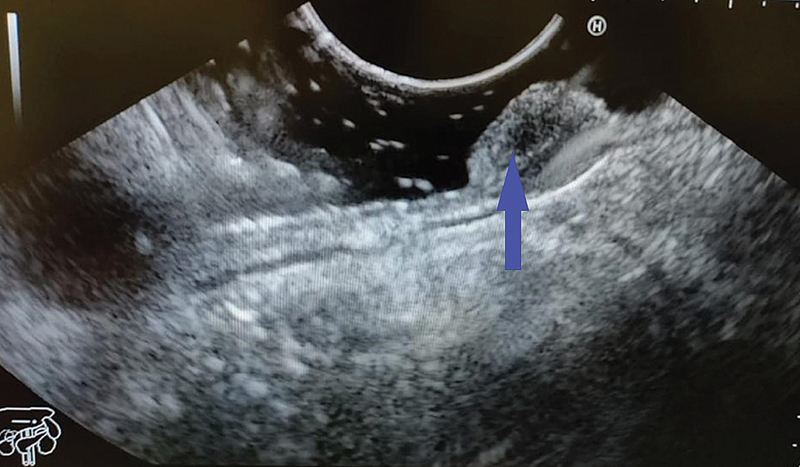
EUS showing a 2.2-cm hyperechoic lesion arising from the submucosal layer with no calcification, cystic change, or ductal structure. EUS, endoscopic ultrasound.
